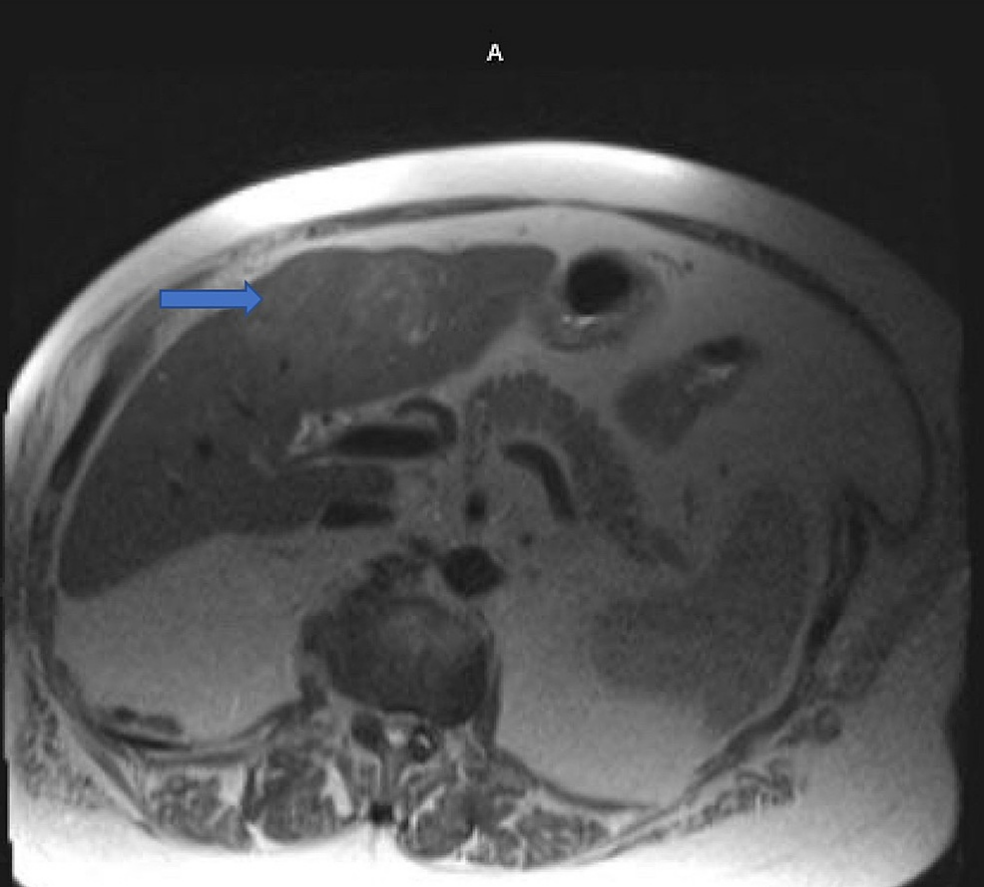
MRI showing a non-uniform enhancement of 6.4 x 8-cm mass in the left lobe of the liver (blue arrow) MRI: magnetic resonance imaging
